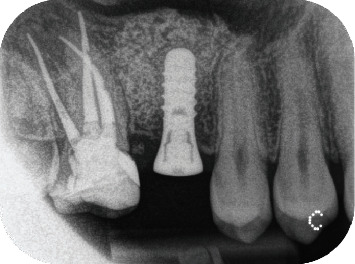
Periapical radiograph obtained immediately after implant placement.
